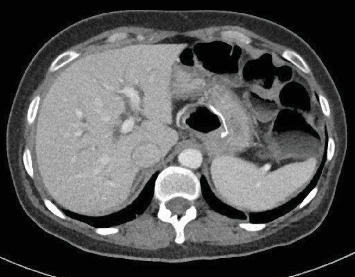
Abdominal computer tomography (CT) showing dilated extrahepatic and intrahepatic bile ducts.
